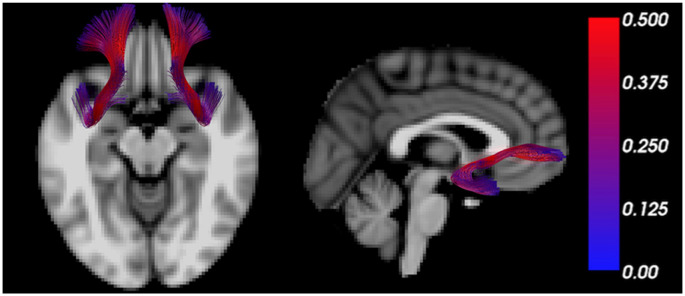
The uncinate fasciculus is a characteristic trajectory connecting the prefrontal cortex (PFC) and amygdala. MRI tractography depicts the uncinate fasciculus overlain on transverse (left) and sagittal (right) T1 weighted sections. The color bar scales the tract by fractional anisotropy (FA) values.
